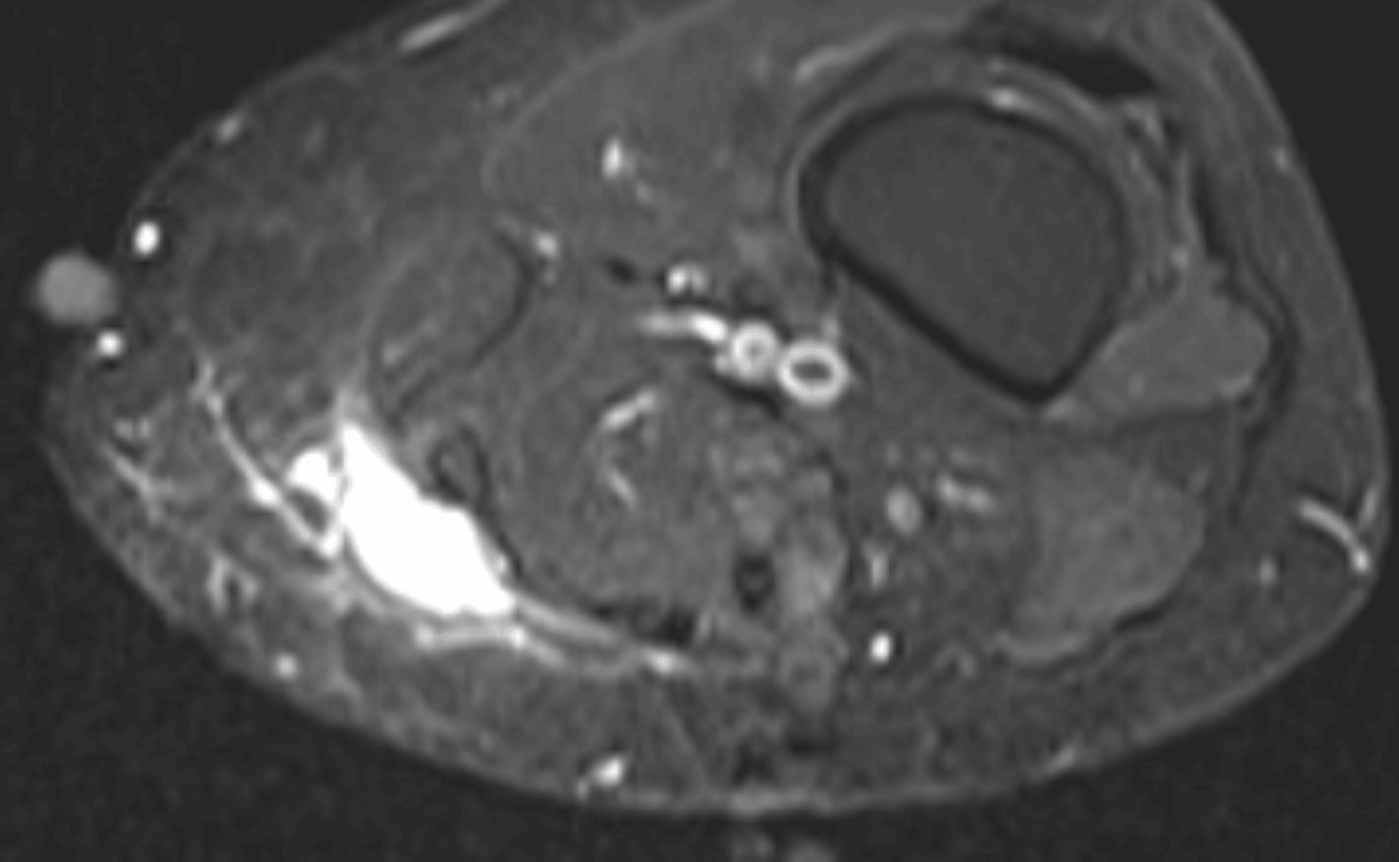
MRI of left thigh mass MRI image showing an ovoid mass along the surface of the superficial fascia abutting the posteromedial aspect of the gracilis and sartorius muscles.
